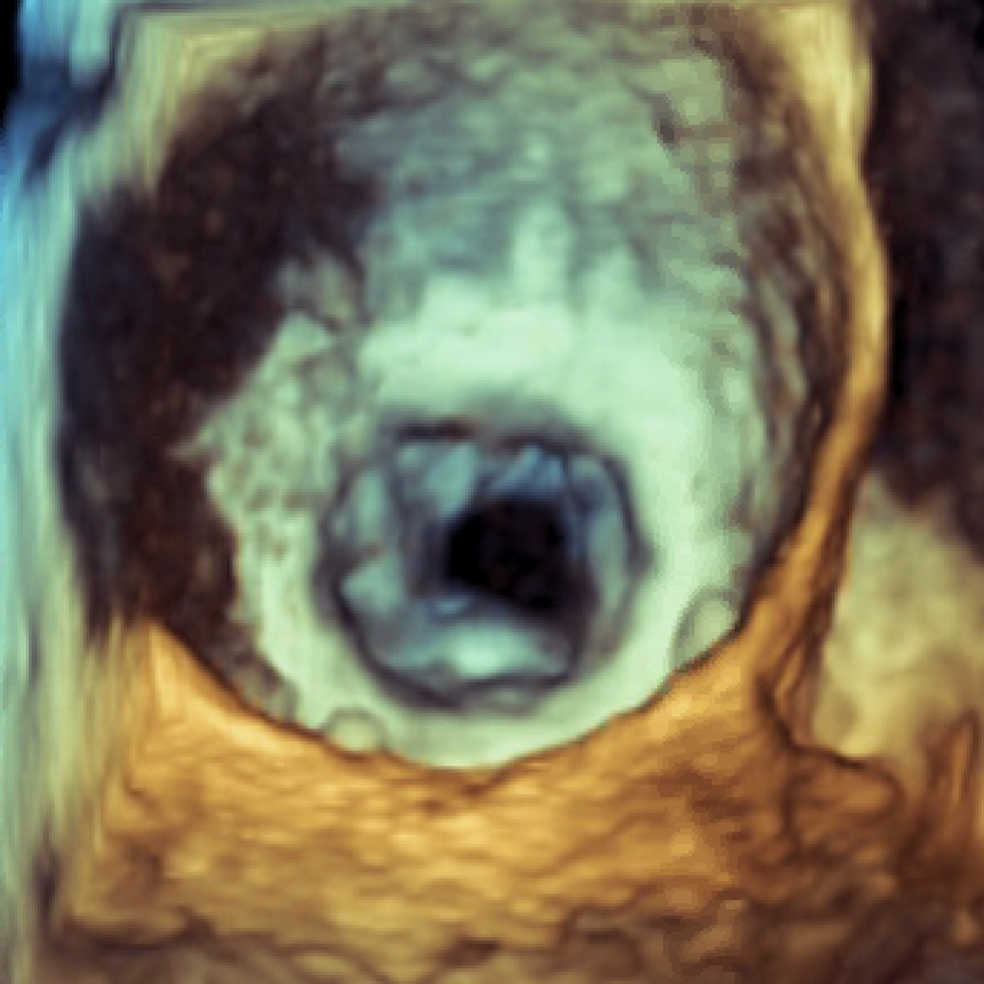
Three-dimensional transesophageal echocardiogram of surgeon's view during diastole Three-dimensional planimeter valve area: 0.77-0.87 cm^2^ (Severe < 1.0 cm^2^).
